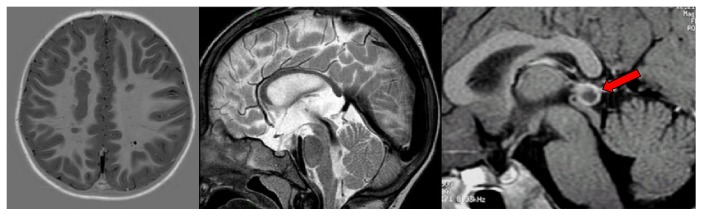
MRI scans: gray matter heterotopia, hypoplasia of the corpus callosum. Red arrow: pineal cyst.
